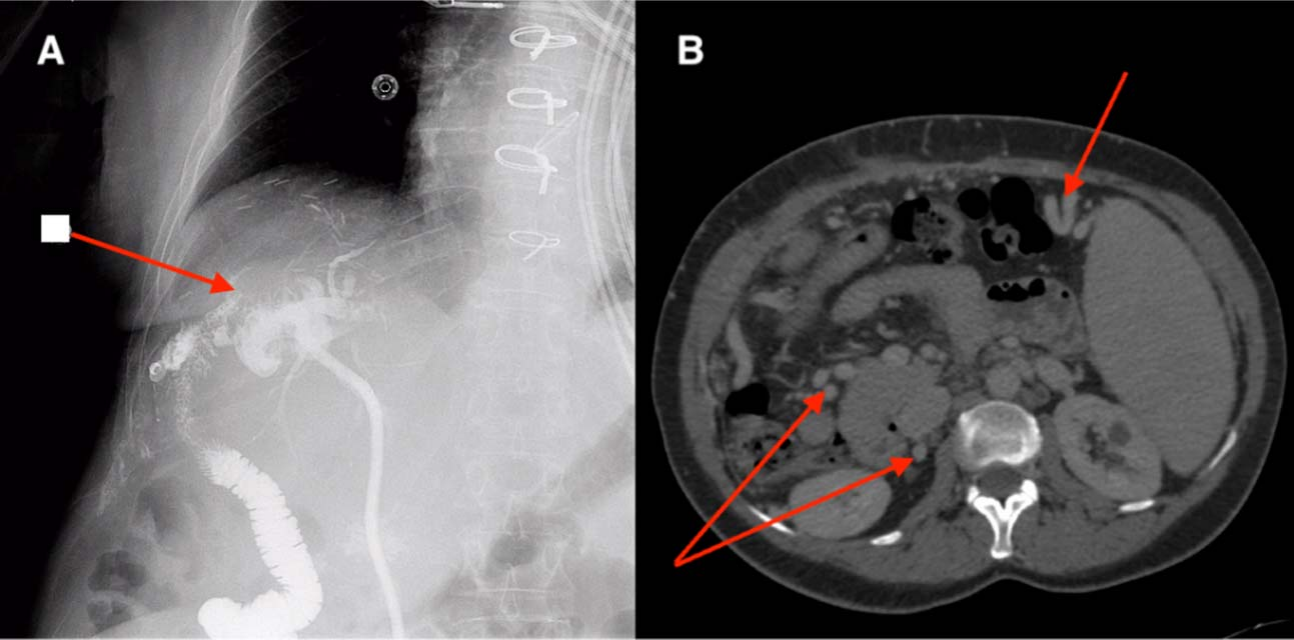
(A) Preoperative cholangiogram depicting filling defect and intrahepatic ducts; (B) computed tomography scan revealing splenomegaly and prominent venous collaterals.
